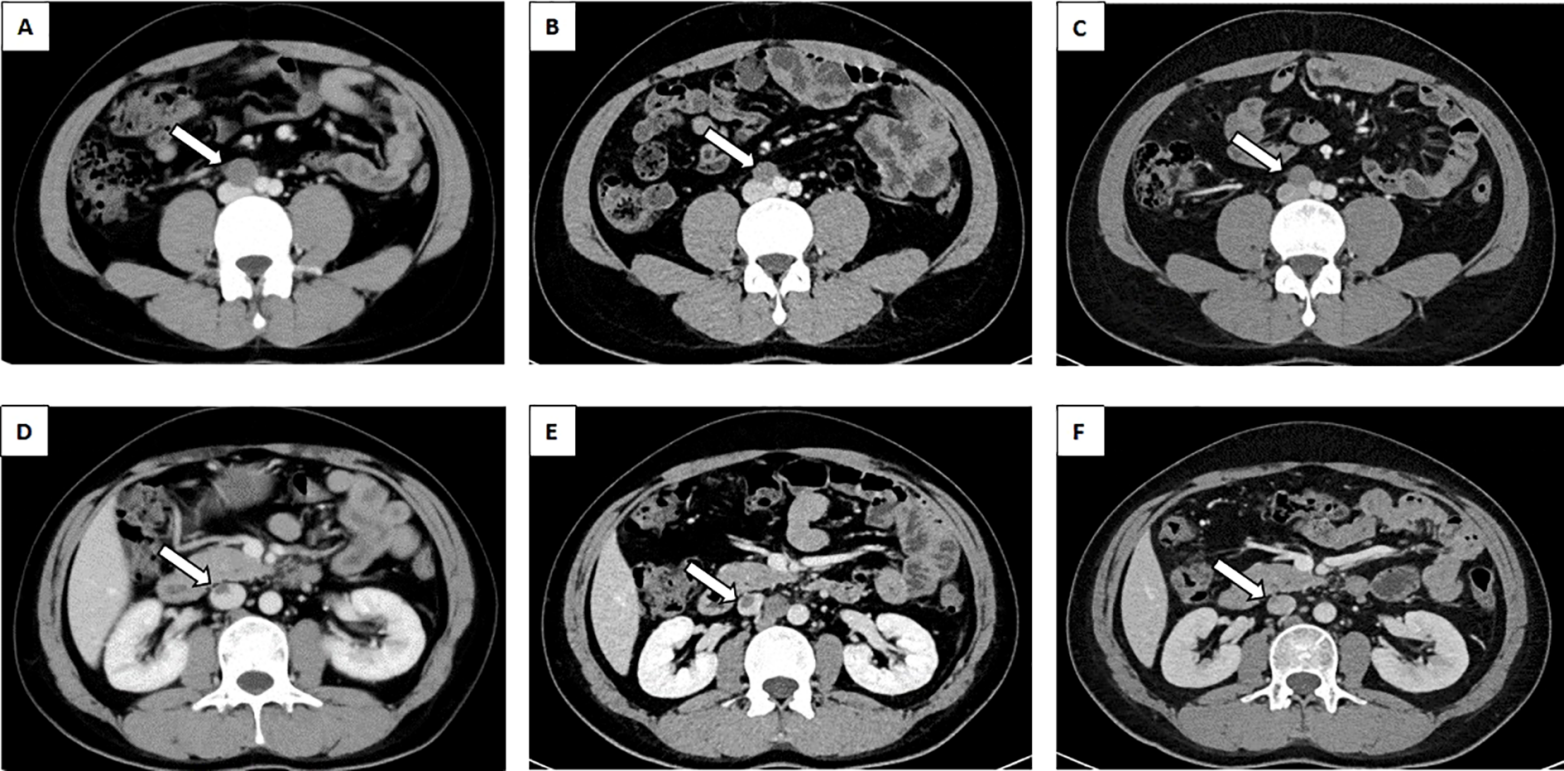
Abdominal CT revealed lymph nodes between the abdominal aorta and IVC. (A) lymph nodes prior to chemotherapy(white arrow). (B) The BEP regime for two cycles of chemotherapy for lymph nodes (white arrow). (C) The TIP regime for one cycle of chemotherapy for lymph nodes (white arrow). Abdominal CT revealed malignant thrombus of the IVC. (D) malignant thrombus of the IVC prior to chemotherapy(white arrow). (E) The BEP regime for two cycles of chemotherapy for malignant thrombus of the IVC (white arrow). (F) The TIP regime for one cycle of chemotherapy for malignant thrombus of the IVC (white arrow). CT, Computed Tomography; BEP, Bleomycin, Etoposide, Cisplatin; TIP, Paclitaxel, Ifosfamide, Cisplatin; IVC, Inferior vena cava.
